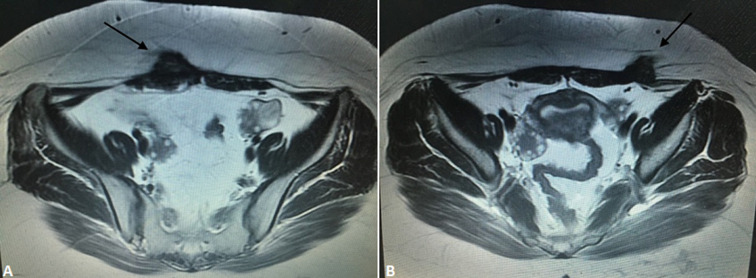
(A, B) MRI images of the masses in the anterior abdominal wall (arrows); the lesions tend to extent to the subcutaneous fat and the right rectus abdominis muscle
